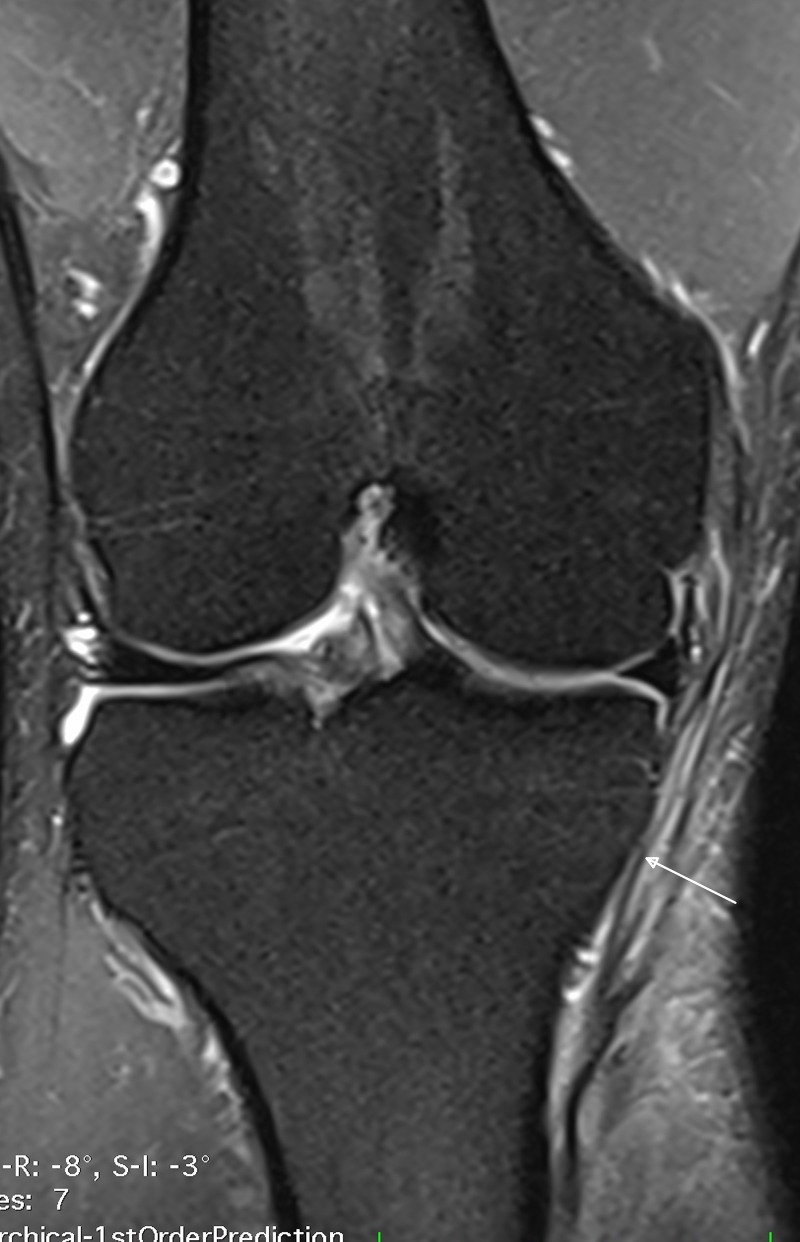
Magnetic resonance imaging of the right knee for patient 1 The T2 weighted image shows the continuity of the fibers of the superficial band of the MCL (white arrow).
